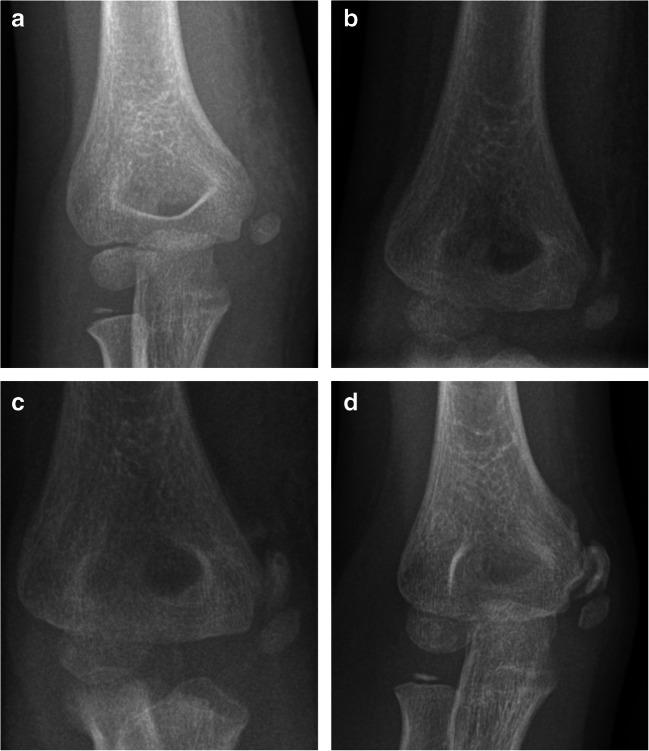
Radiographs of the right elbow of a 7-year-old girl in anteroposterior projection after elbow trauma. a Initial radiograph showing the ossification center of the medial epicondyle located somewhat distant from the humerus, consistent with an avulsion of the epicondyle. b The follow-up radiograph after 7 days shows a half-moon-shaped ossification zone between the humerus and the still distant medial epicondyle leading to a multipartite appearance of the medial epicondyle. c Increasing size of the now ovoid callus next to the ossification center of the medial epicondyle after 14 days. d After 4 months, the posttraumatic medial epicondyle remains a multipartite appearance with further progression of the callus
